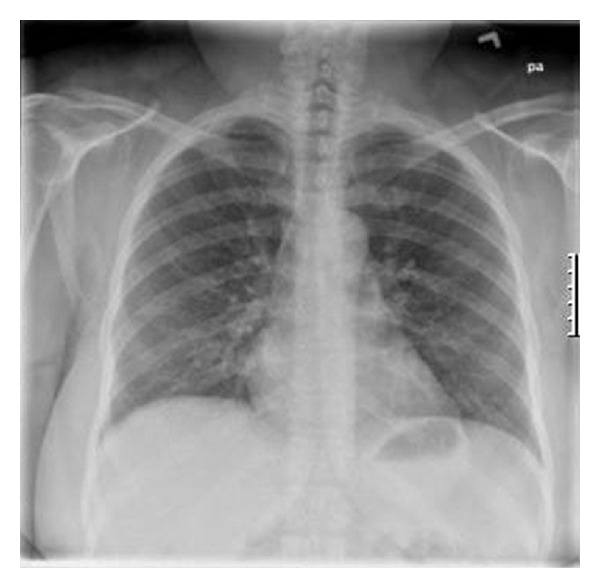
Chest radiograph on day no. 3, a near normal study with marked radiological resolution of interstitial disease.
